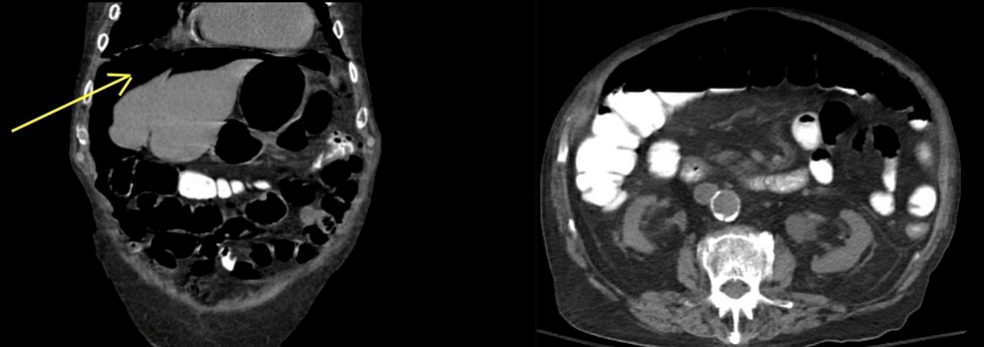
CT abdomen/pelvis showing large pneumoperitoneum
